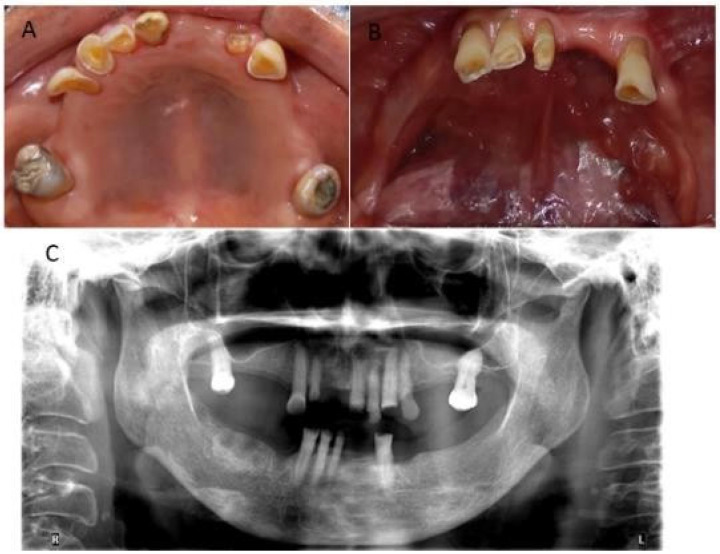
Upper (A) and lower (B) occlusal intraoral photography and (C) panoramic radiograph.
